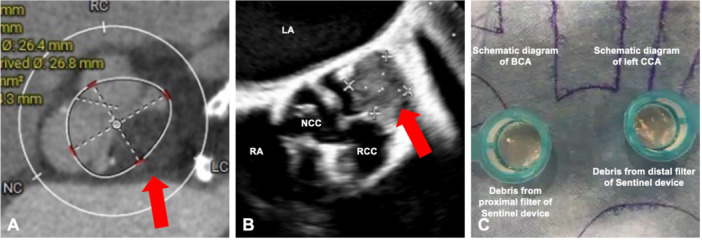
Patient 3. Aortic root thrombus in the LCC [red arrow] was found on (A) computed tomography and (B) transoesophageal echocardiography (TEE). (C) Multiple debris up to 4 × 2mm were captured by SENTINEL embolic protection device. [Color figure can be viewed at wileyonlinelibrary.com]
